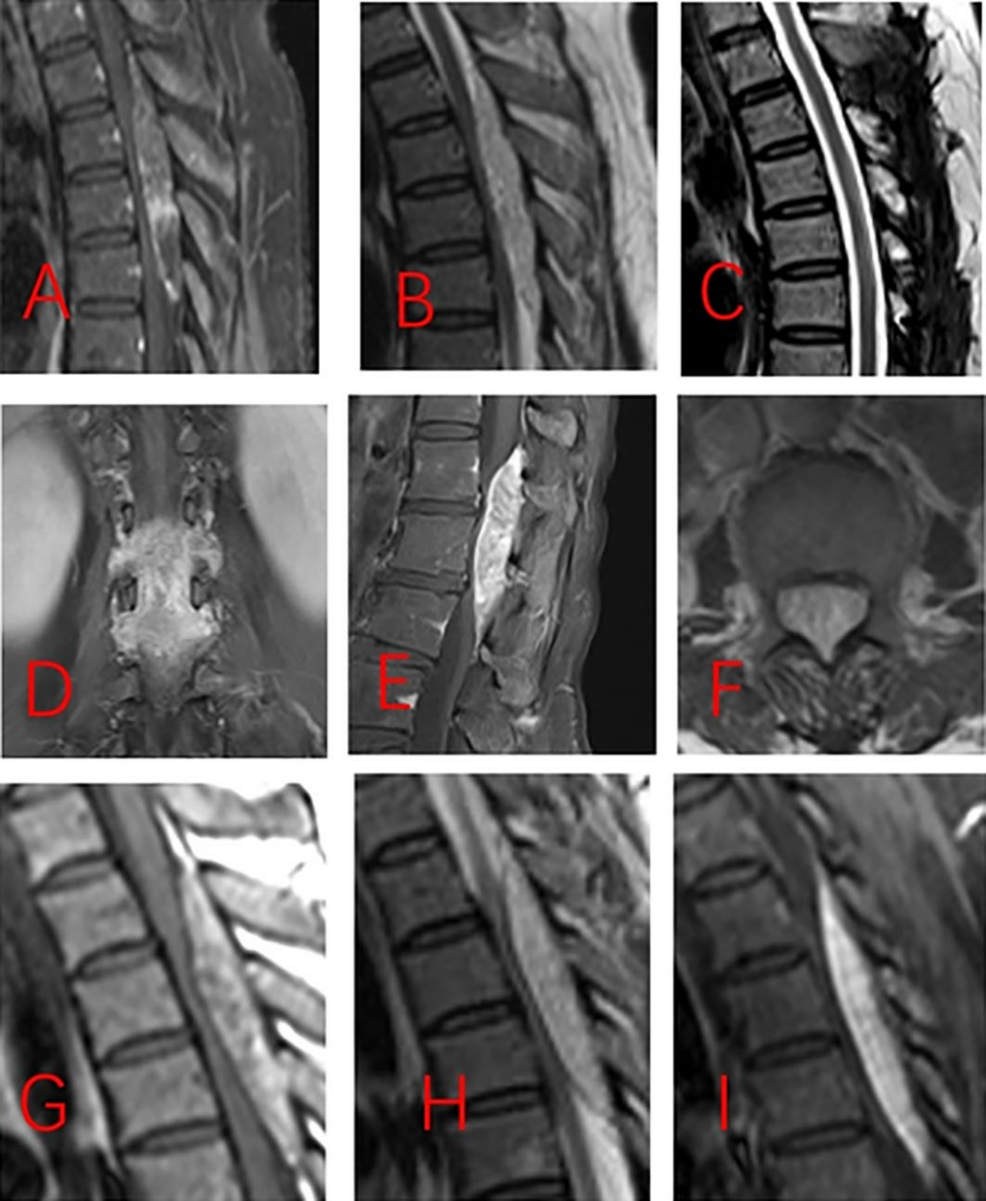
MRI of the three patients from our institution. (A) and (B): First patient’s preoperative T1WI and T2WI images. C: First patient’s postoperative T2WI images. (D), (E), and (F): The second patient’s preoperative T1 enhancement coronal, sagittal, and axial images. (G), (H) and (I): The third patient’s preoperative T1WI, T2WI and T1 enhancement images.
